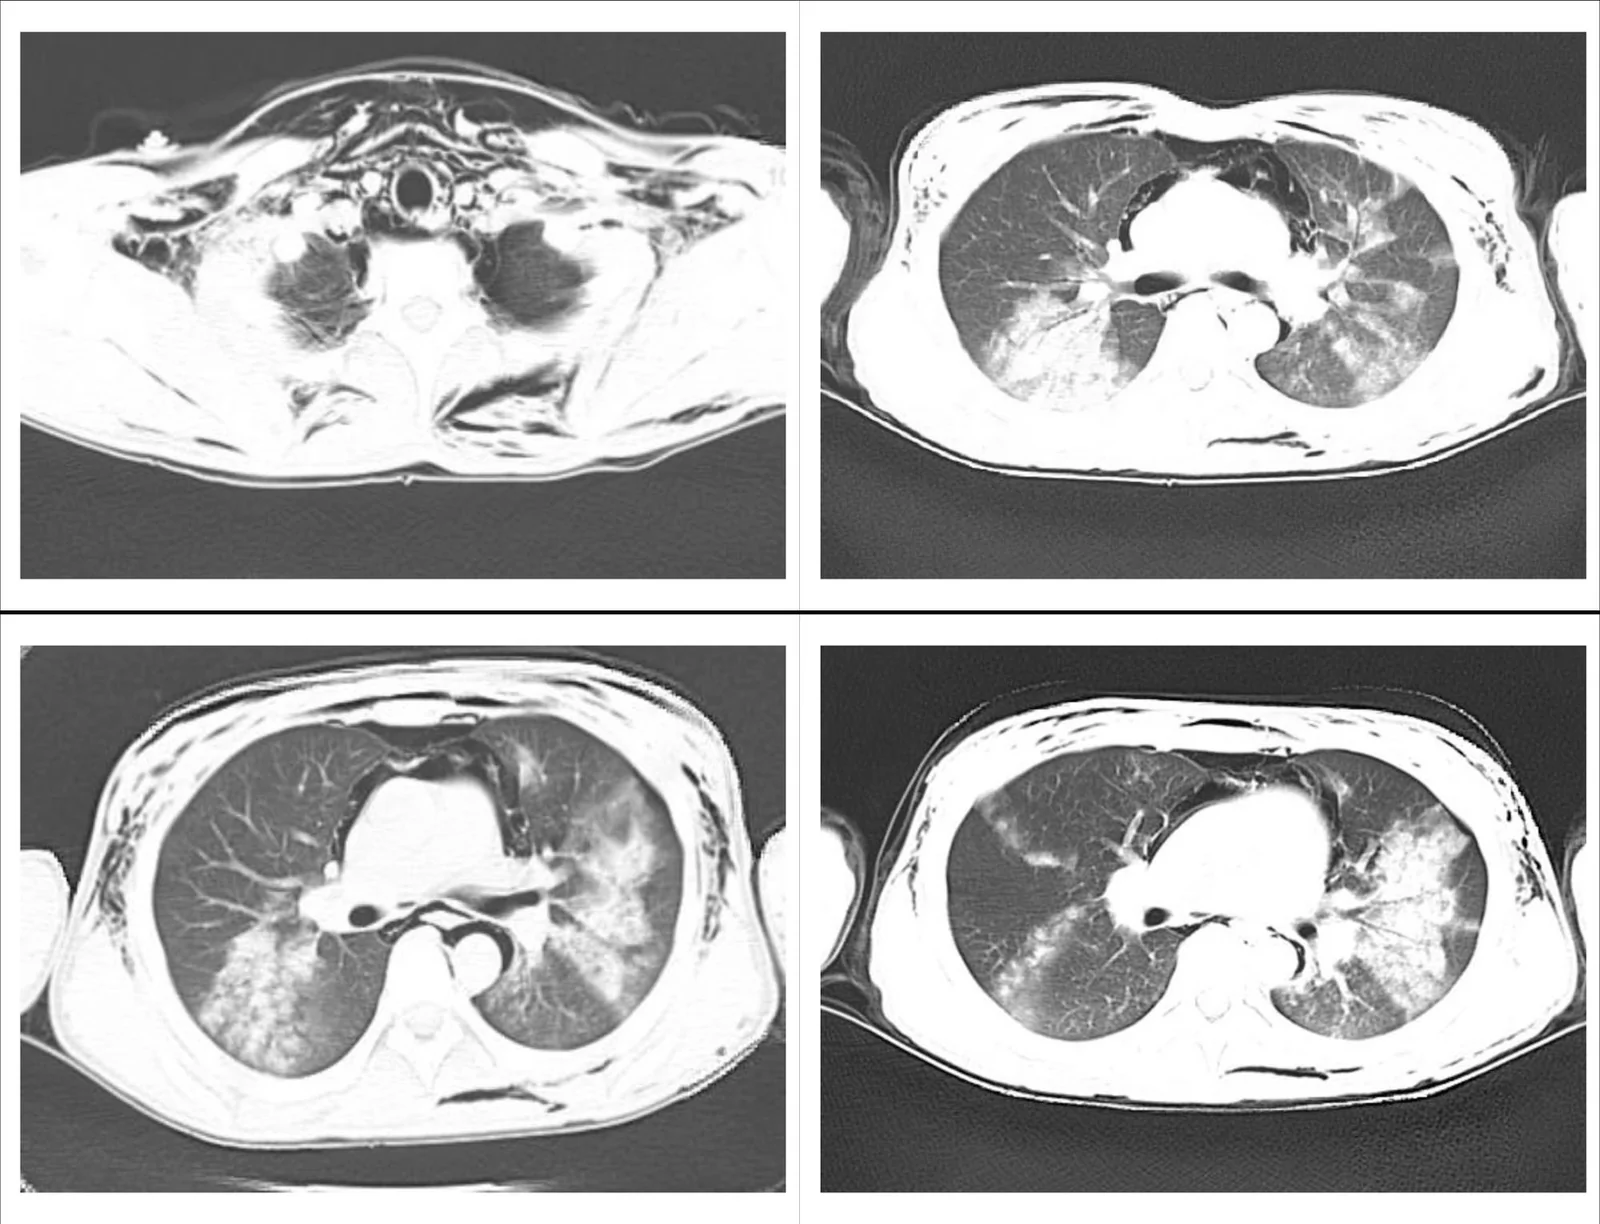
CT performed after HBOT demonstrated bilateral pulmonary exudative changes, subcutaneous emphysema, pneumomediastinum, and pneumothorax. CT = computed tomography, HBOT = hyperbaric oxygen therapy.
